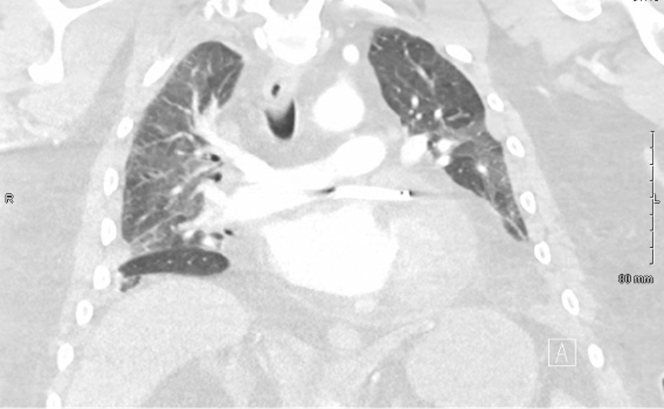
Chest Computed Tomography Angiography Demonstrating No Pulmonary Embolism Coronal contrast-enhanced imaging shows no filling defect to the level of the proximal segmental pulmonary arteries. Small bilateral pleural effusions are present with associated lower lobe subsegmental atelectasis. No pulmonary mass or pneumothorax is identified.
